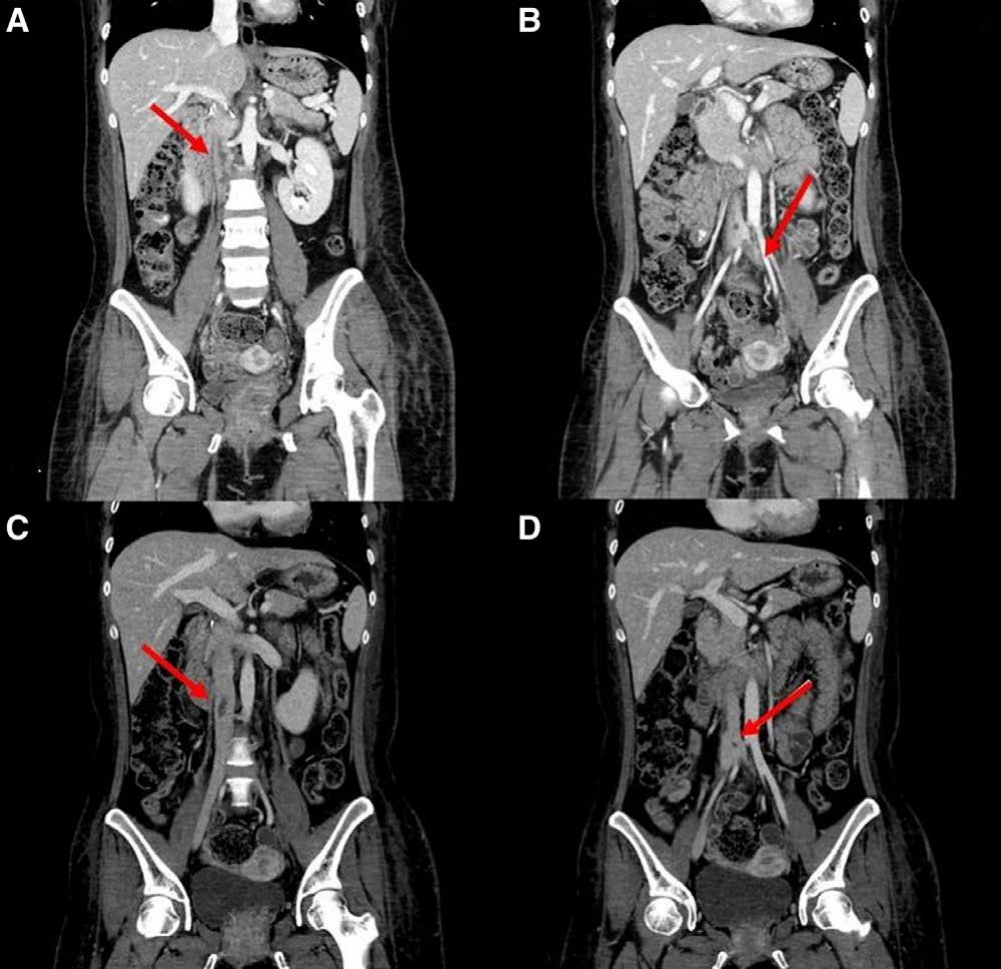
(A, B, C, D) The follow up abdominal CT scans. The red arrow lines indicate a thrombus in the IVC. CT scans were obtained 2 months after admission and show a reduced burden of the IVC thrombus. CT = computed tomography, IVC = inferior vena cava.
